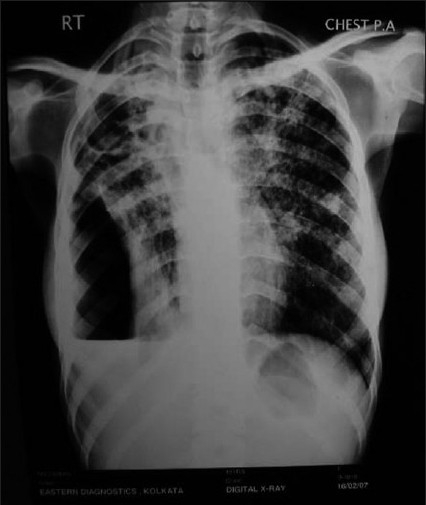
Chest X-ray showing right-sided pyopneumothorax with bilateral parenchymal lesions
